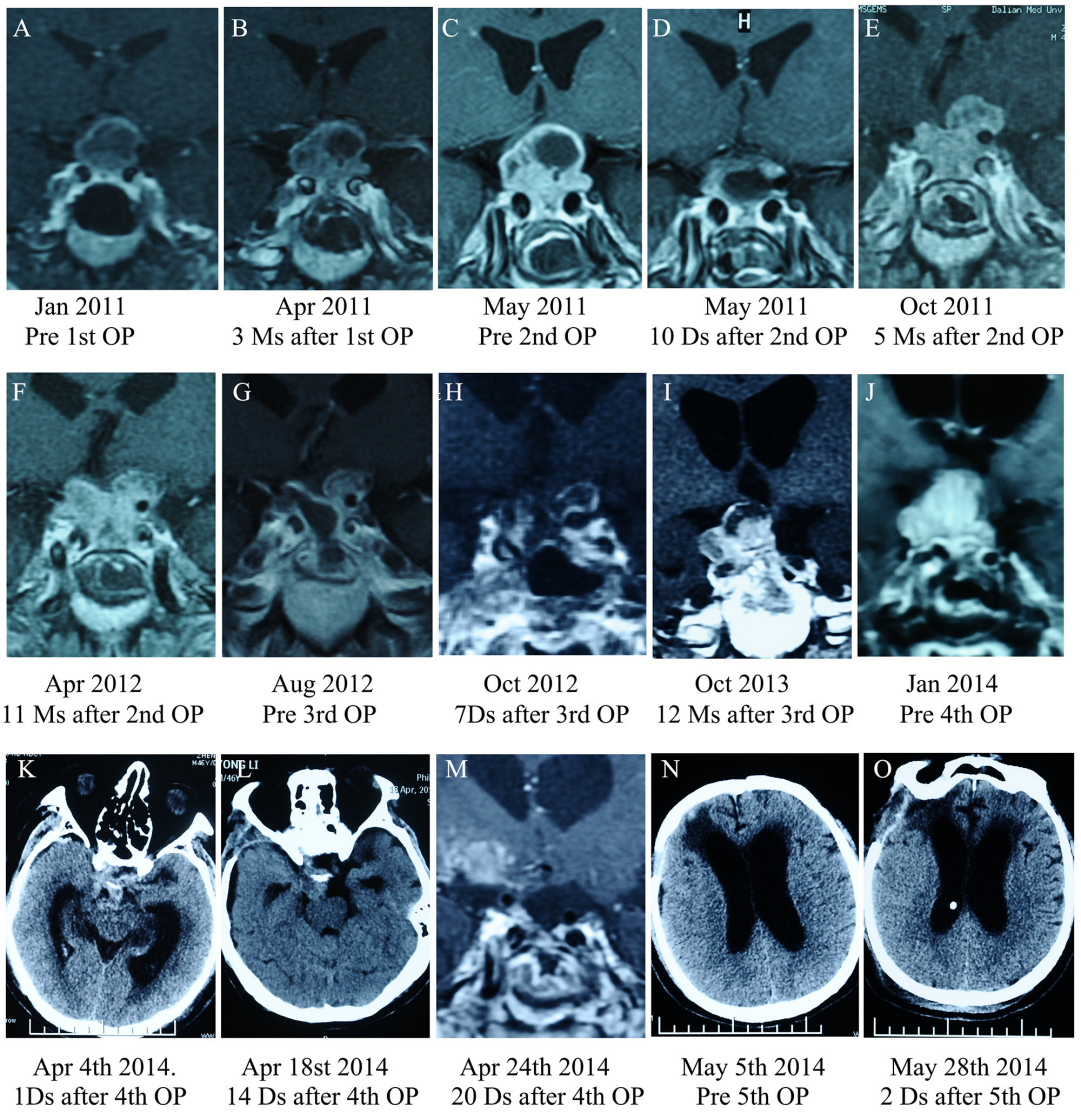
Pituitary MRI images for the case three patient A. MRI images at initial diagnosis. B. 3 months after the first operation. C. Pre-operative MRI image prior to the second TSS. D. 10 days after the second operation. E. 5 months after the second operation. F. 11 months after the second operation. G. Before the third operation. H. 7 days after the third operation. I. 1 year after the third operation. J. Before the fourth operation. K, L and M. CT scan after the fourth operation showing subtotal tumor removal. N. In May 2014, the patient presented with hydrocephalus, and a ventriculoperitoneal shunt was implanted; hydrocephalus subsequently improved O.
